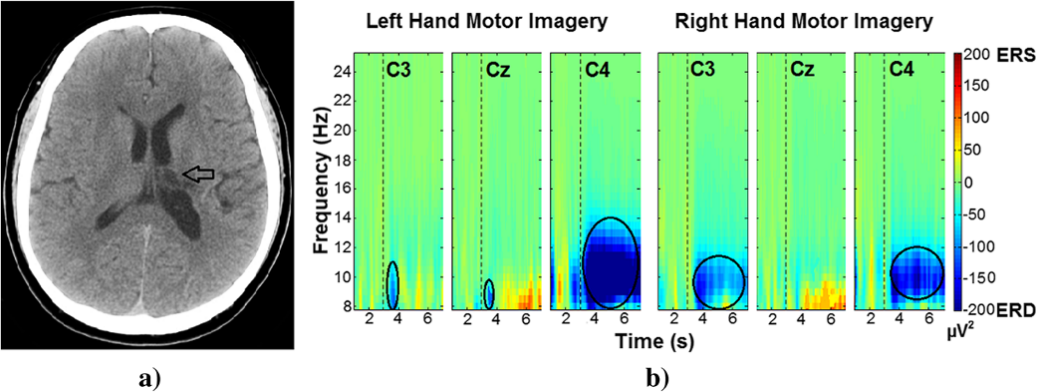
Male patient CT image and averaged TFRs. a) Representative image of the male patient’s CT (Computed Tomography). The black arrow indicates the location of the residual injury. b) Average TFR for left and right hand (affected limb) motor imagery in 8 Hz to 25 Hz from 1 s to 7 s. The dashed line at 3 seconds indicates the motor imagery onset. The black circles highlight the generated ERD.
